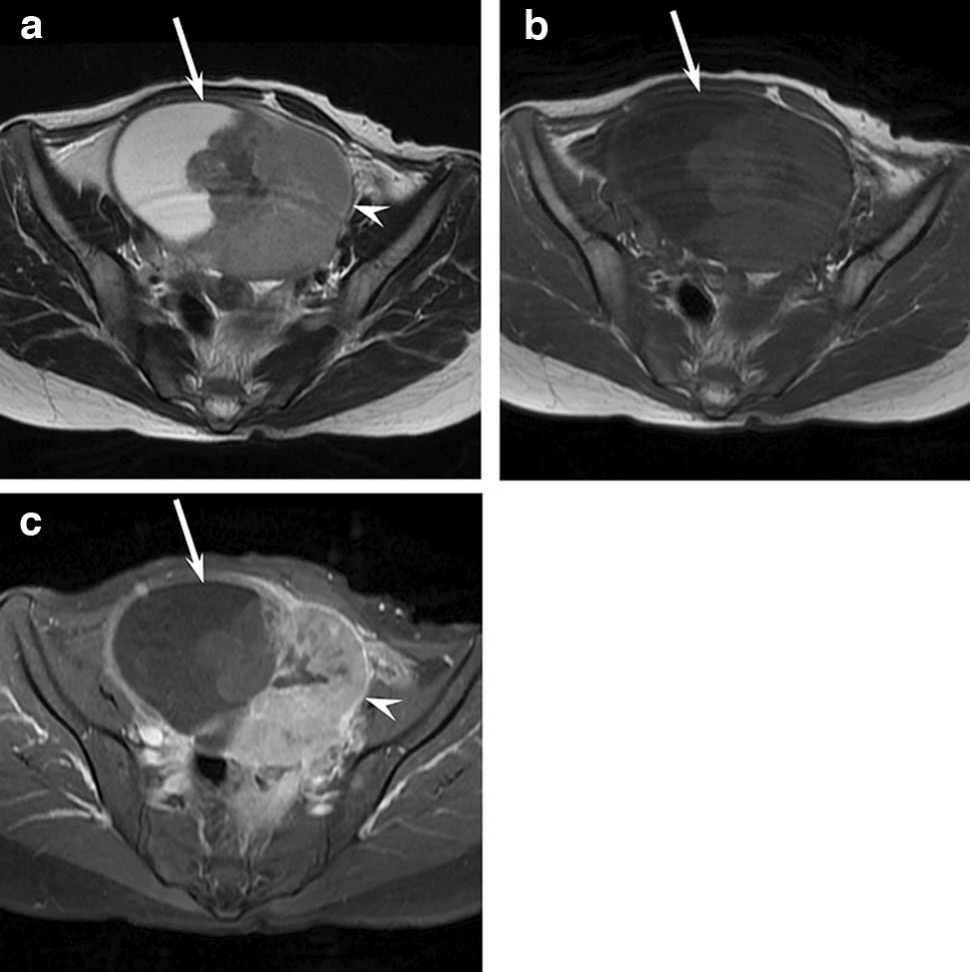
A 49-year-old woman with MT-MCT (stage IIA). a. Axial T2-weighted image showing a cystic mass (arrow) with endophytic sessile solid components (arrowhead). b. Axial T1-weighted image showing a cystic mass (arrow) without fatty components. c. Axial fat-suppressed contrast-enhanced T1-weighted image showing a cystic mass (arrow) with endophytic sessile solid components (arrowhead)
